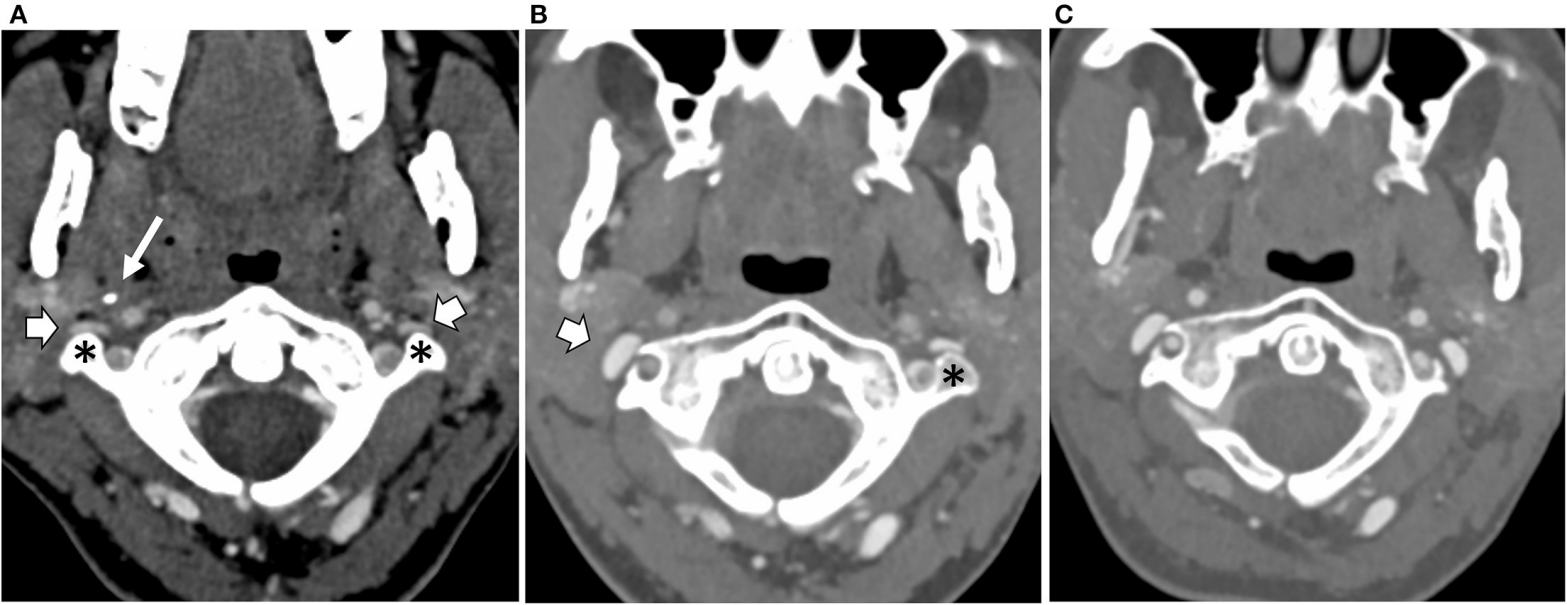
Axial CT scans at the level of the C1 vertebra and odontoid peg following intravenous contrast injection, (A) at the baseline, (B) following resection of the right styloid and C1 transverse processes, and (C) following resection of the left C1 transverse process. The baseline scan (A) shows narrowing and flattening of both internal jugular veins (block arrows) as they pass in front of the transverse processes of C1 (asterisk) on either side. The styloid process of the skull (thin arrow) can be seen on the right side. (B) Following the right transverse process and styloid resections, the right internal jugular vein (block arrow) has expanded into the space created by the surgery. (C) Following left transverse process resection, the left internal jugular vein has now also expanded.
